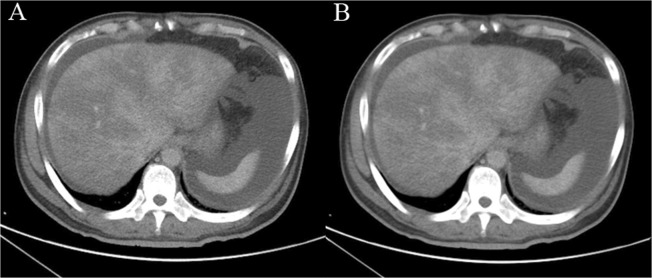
(A) 49-year-old man was diagnosed with PA-SOS and underwent contrast-enhanced CT. CT images were denoised using the anisotropic diffusion method; (A) original and (B) denoised.
